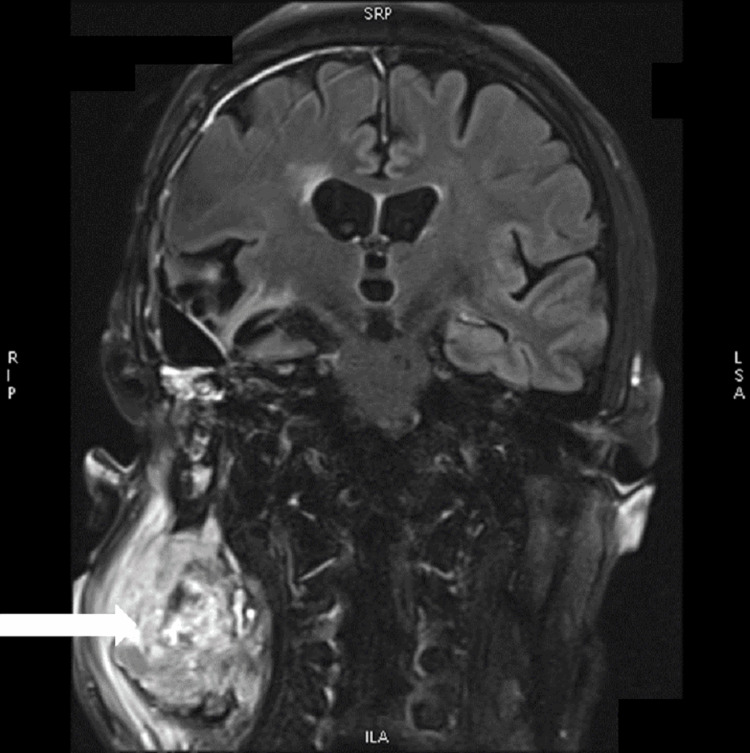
COR T2 TIRM postcontrast MRI of the brain showing the persistent postoperative changes in the right temporal lobe and a big heterogenous contrast-enhancing tumor in the neck on the right side (arrow) COR T2 TIRM: coronal transverse relaxation time turbo inversion recovery magnitude; MRI: magnetic resonance imaging
